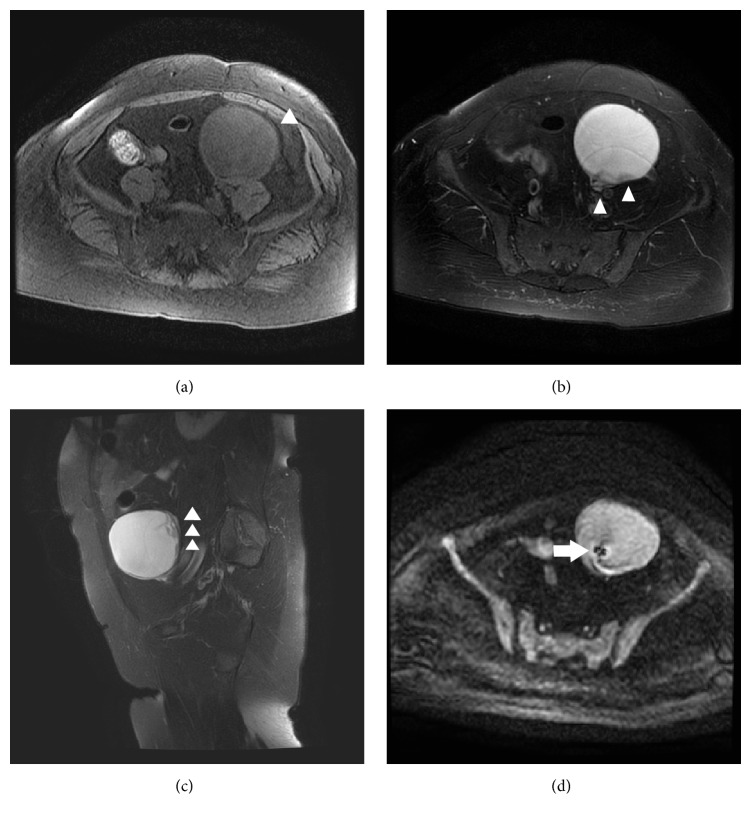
Magnetic resonance imaging findings. (a) T1-weighted transverse image, revealing an ovarian mature cystic teratoma (arrowhead). T2-weighted transverse (b) and sagittal (c) images, demonstrating heterogeneous signal intensity at the posterior wall (arrowheads). (d) Diffusion-weighted transverse image, demonstrating low signal intensity at the septum (arrow).
